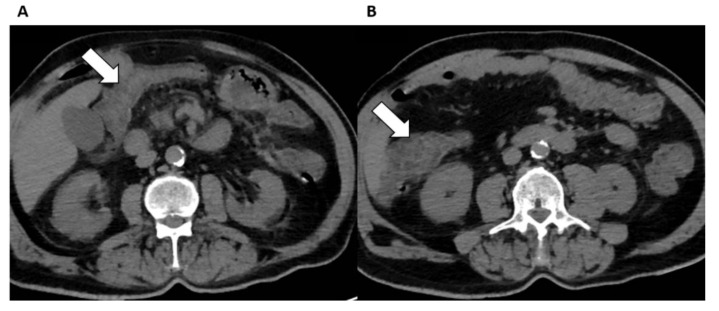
Intestinal findings in a COVID-19 patient admitted to the Emergency Department for abdominal pain. (A,B) Unenhanced axial abdominal CT images show bowel wall thickening in the transvers colon (arrows) with adjacent fat stranding consistent with intestinal involvement with ischemic lesions.
